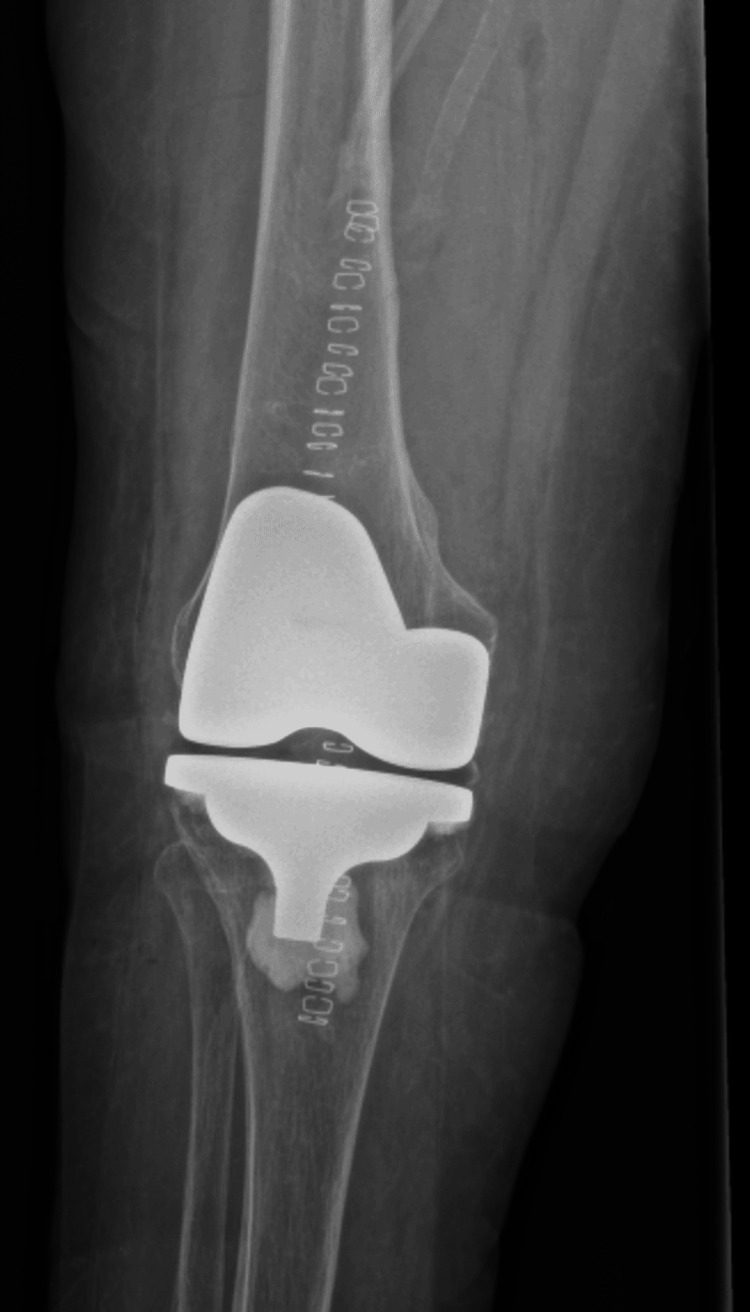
Postoperative plain radiography, AP, non-weight bearing view, demonstrating new prosthesis.
